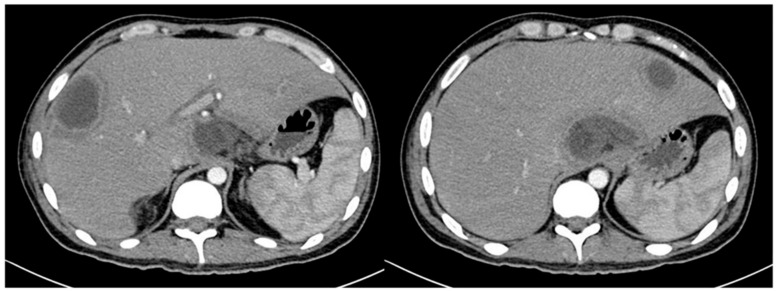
Abdominal CT scan with intravenous contrast, which shows: Diffuse and poorly defined hypodense lesions, one located in the liver segment II measuring 3.4 cm, another located in the caudate lobe measuring 7 cm, and another located in the hepatic segment VII with extension to segment VI measuring 7 cm; consistent with liver abscesses.
